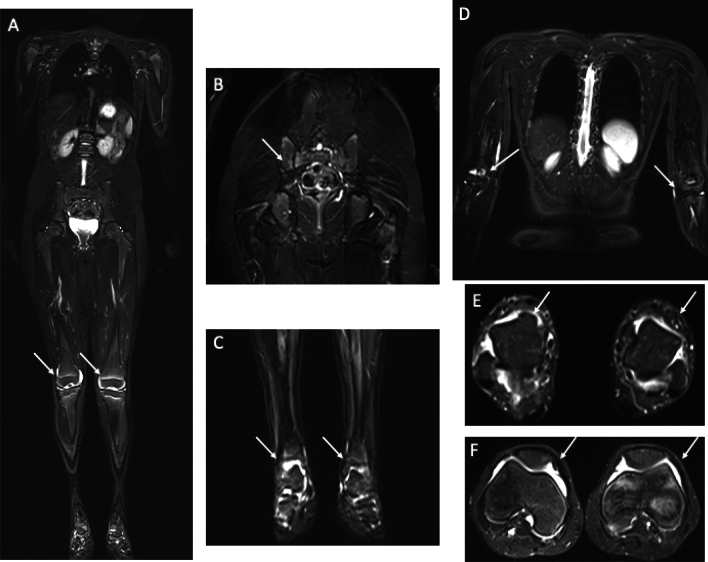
WB-MRI in a 9-year-old girl with oligoarticular juvenile idiopathic arthritis (JIA). Whole-body (a) and STIR images in coronal (b, c and d) and axial (e and f) planes show right sided sacroiliitis (b), synovitis in bilateral knees (a and f), ankles (c and e) and elbows (d) with some spots of BME
